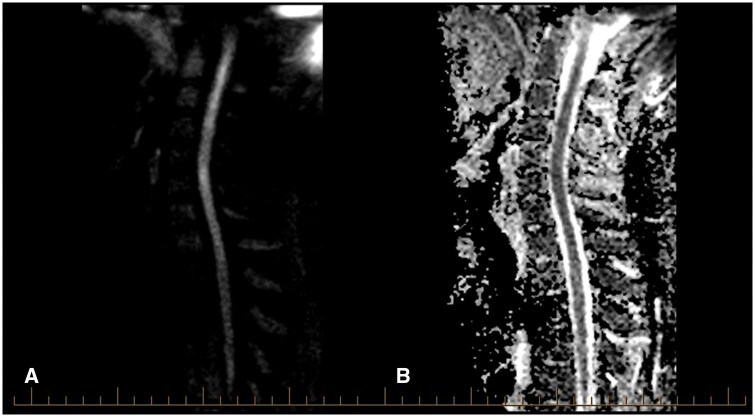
(A) Sagittal Diffusion Weighted MRI demonstrated a spinal cord infarct. (B) Matched sagittal MRI ADC demonstrating CSI.
